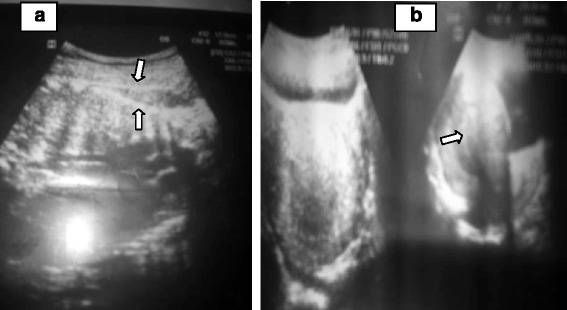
Ultrasound images of abdominal pregnancy. a The fetal spine (*lower arrow*) can be seen just under abdominal wall echo (*upper arrow*) without intervening myometrial tissue. b An empty uterus
